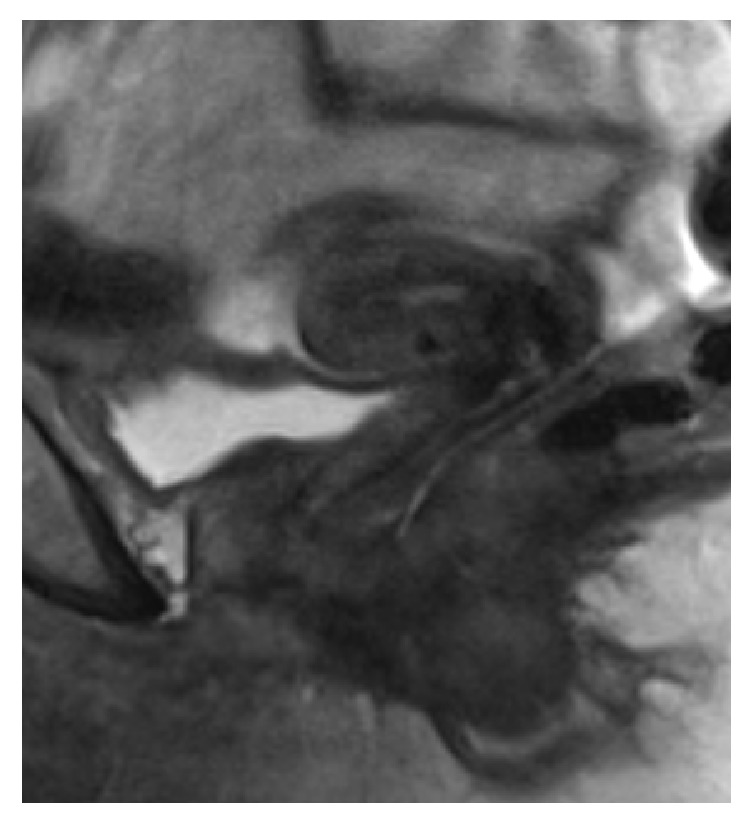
Sagittal MRI in Case 1 showing uterus, cervix, and vagina after menopause.
